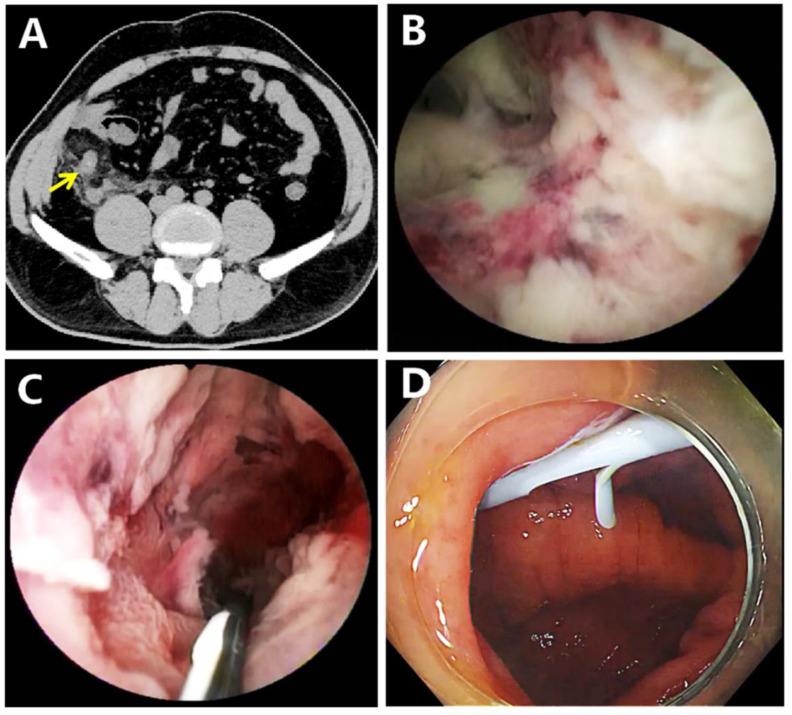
(A) The computed tomography revealed that the appendix was significantly thickened, along with peripheral inflammatory exudation (yellow arrow). (B) Cholangioscope showed that the appendiceal mucosa appeared dark brown. (C) A guidewire was implanted into the lumen of the appendix. (D) A plastic biliary stent was placed.
